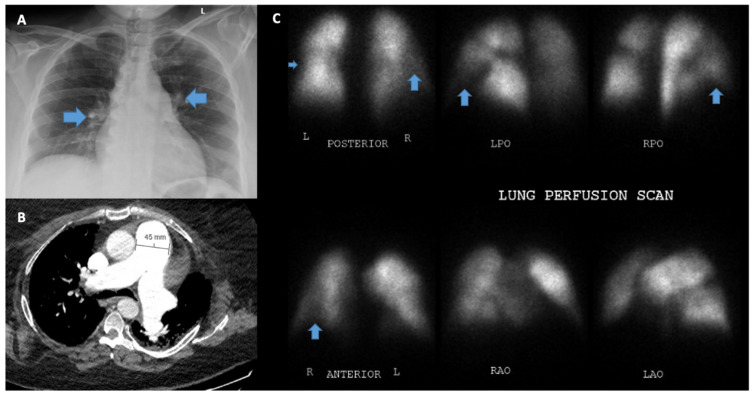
Thoracic imaging of pulmonary hypertension. (A) Chest X-ray with prominent pulmonary artery markings. (B) CT chest with dilated main pulmonary artery, which is larger than the ascending aorta. (C) Perfusion scan with arrows marking abnormal perfusion defects.
